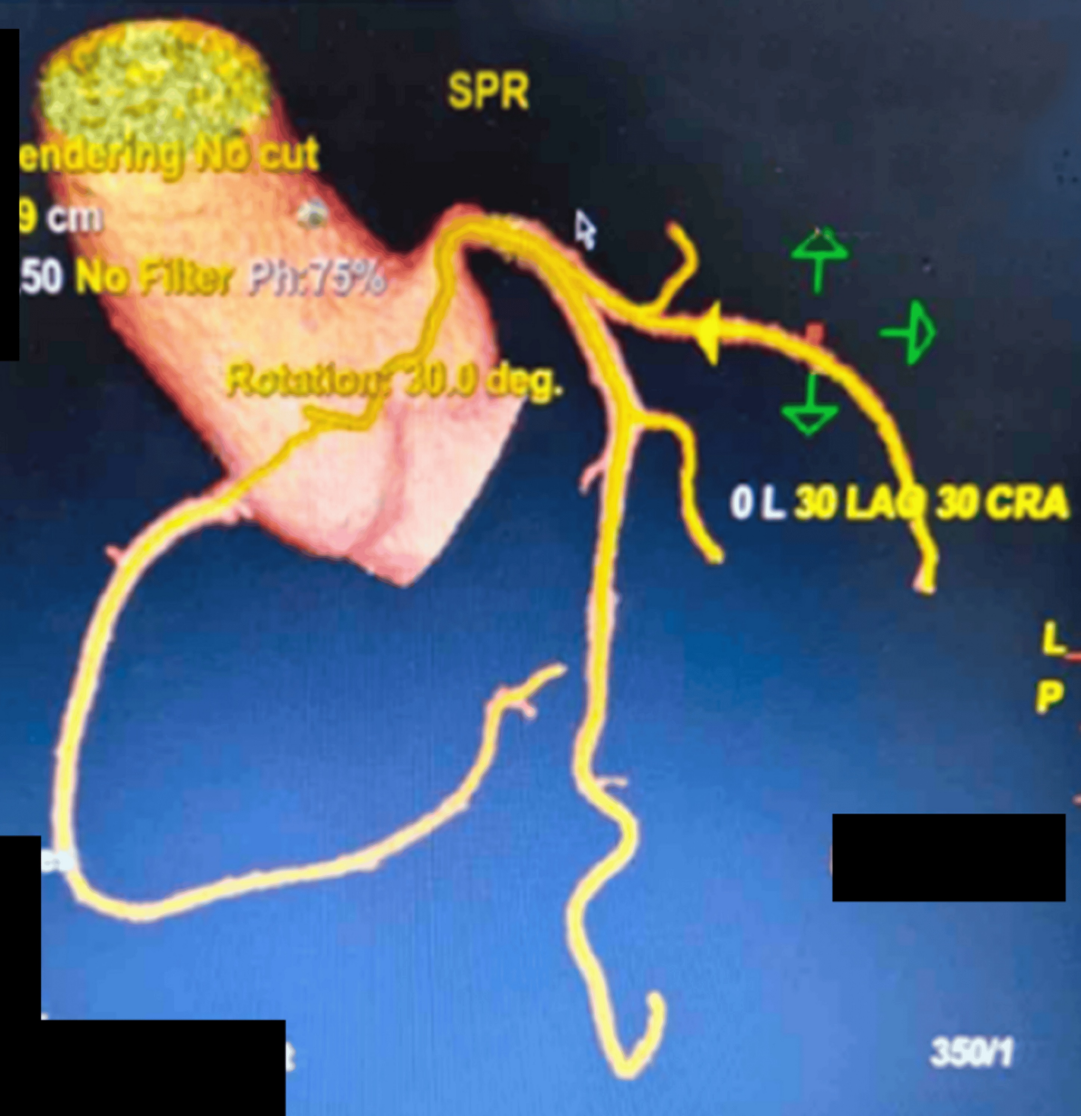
CT coronary angiogram: postoperative three-dimensional reconstruction of the reimplanted RCA in an isolated aortic view CT, computed tomography; RCA, right coronary artery
